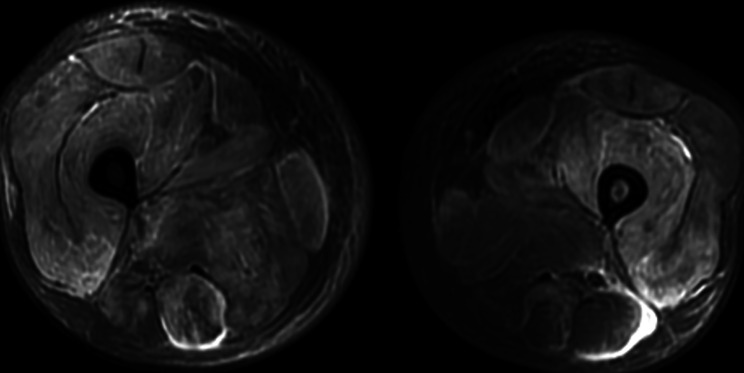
Representative magnetic resonance imaging (MRI) images of diffuse edema in the thigh muscle. Bilateral edema of all thigh muscles and intermuscular fascia and subcutaneous soft tissue edema
